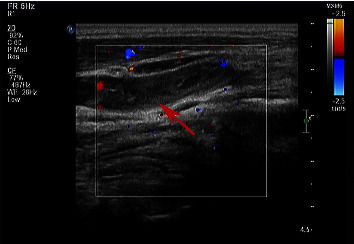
Ultrasonography of the cervical mass showed the left external jugular vein is widened which appears to be filled with no echo in its main trunk.
